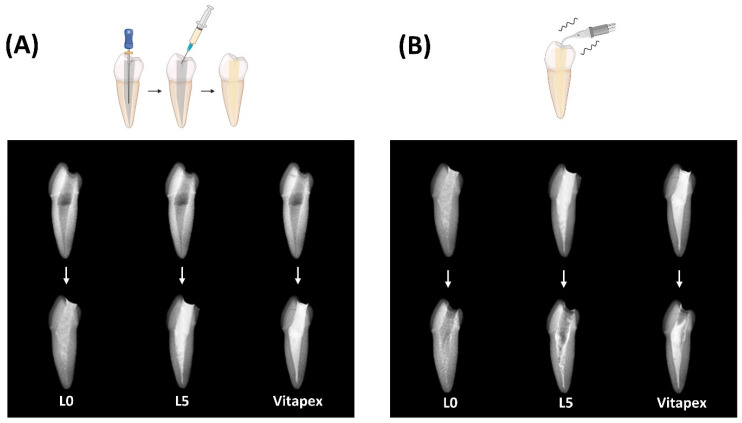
Root canal-filling ability and paste removal test for paste. (A) Radiographs of each tooth after root canal filling with each paste following the preparation of the resin tooth. (B) Radiographs of each tooth after washing with an ultra scaler for 10 s under the same intensity and frequency conditions to confirm paste removability.
